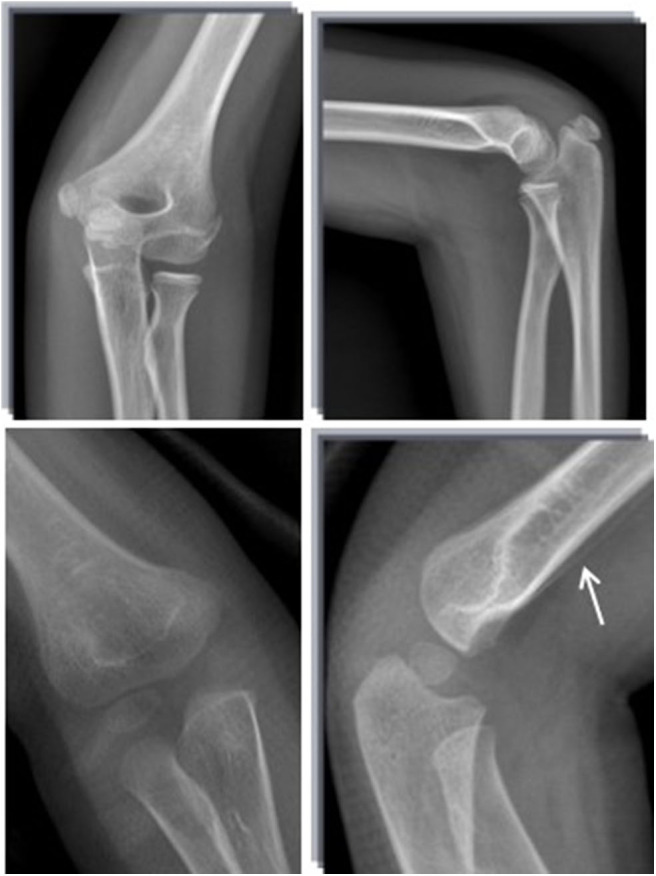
Standard radiograph in AP and LL projections. Fractures were not visible at the first visit. After 3 weeks, a healing line (arrow) could be seen clearly.
